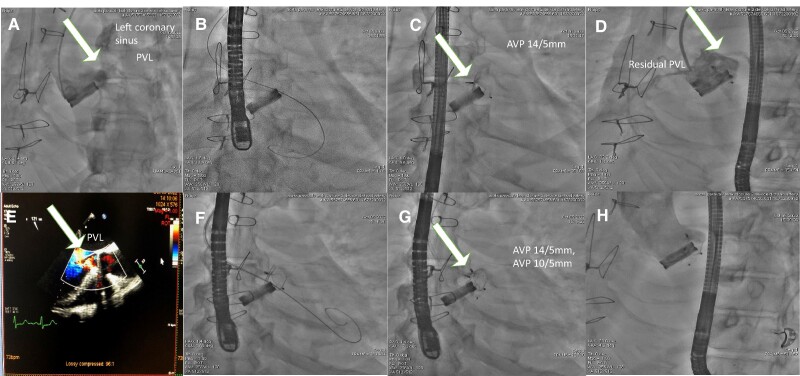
(*A*) Aortography revealing a large-volume paravalvular leak between the outer mechanical valve body and the annulus. A white arrow points at the leak. (*B*) The Confida wire position is carefully checked from different projections to make sure that it is outside the mechanical aortic valve ring. (*C*) A 14/5 mm AVPIII device is released and does not influence valve leaflet motion. A white arrow points at the device. (*D*) Control angiography reveals a decreased but still non-negligible volume paravalvular leak. A white arrow points at the residual leak after AVP 14/5 mm placement. (*E*) Intraoperative transoesophageal echocardiography after the first AVPIII (14/5 mm) release demonstrates still a large-volume leak. (*F*) A careful position check of the Confida wire demonstrates a good position of the wire in between the mechanical valve ring and the prior-implanted AVPIII (14/5 mm). (*G*) The picture after deployment of the second AVPIII (10/5 mm). A white arrow points at the double AVP devices. (*H*) Final angiography demonstrates a small-volume paravalvular leak.
